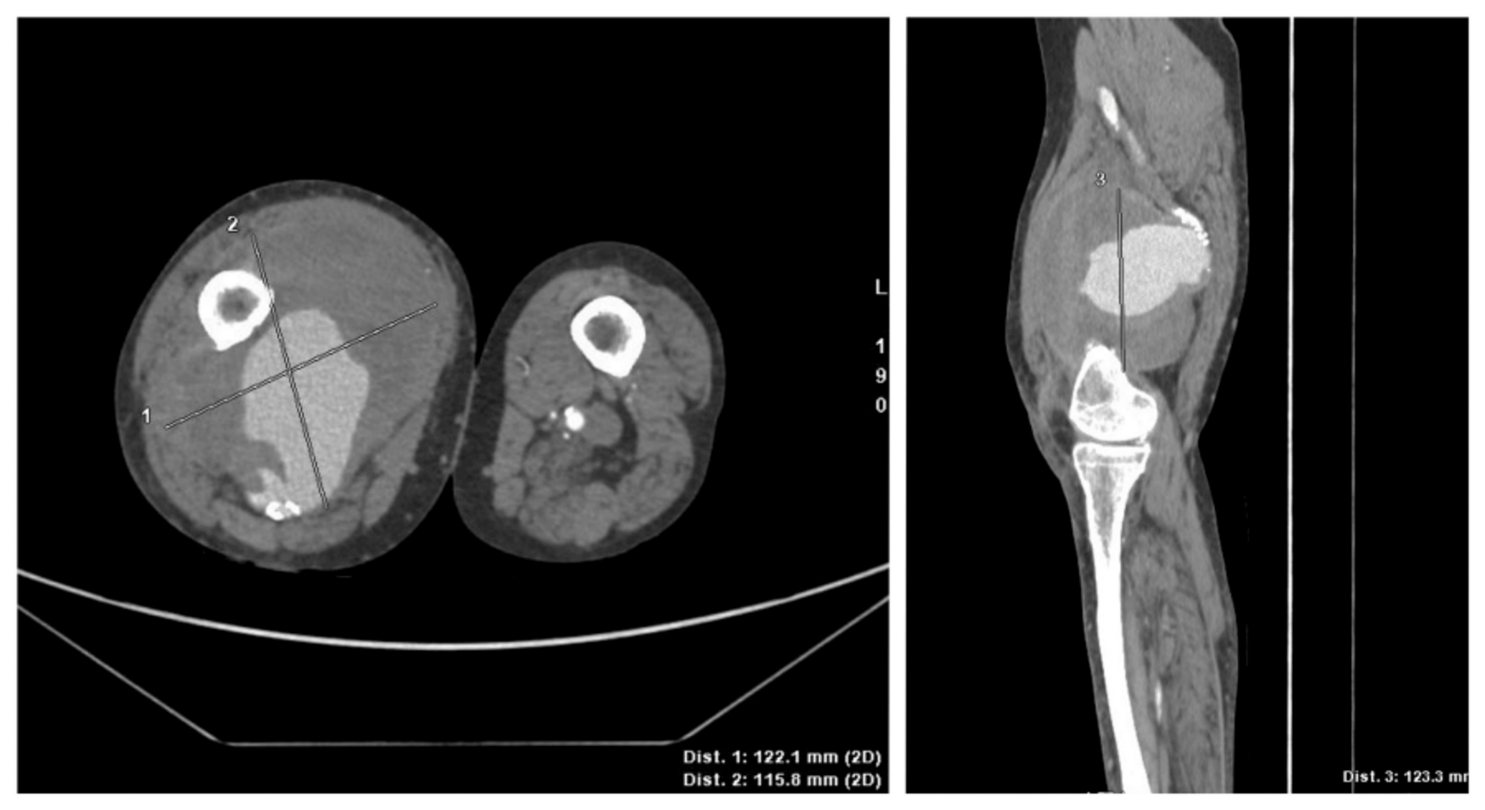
CTA demonstrated a large pseudoaneurysm from the right SFA, measuring 12.2 cm x 11.6 cm x 12.3 cm. Severe arterial calcification at the rupture site was demonstrated. CTA: computed tomography angiography; SFA: superficial femoral artery
